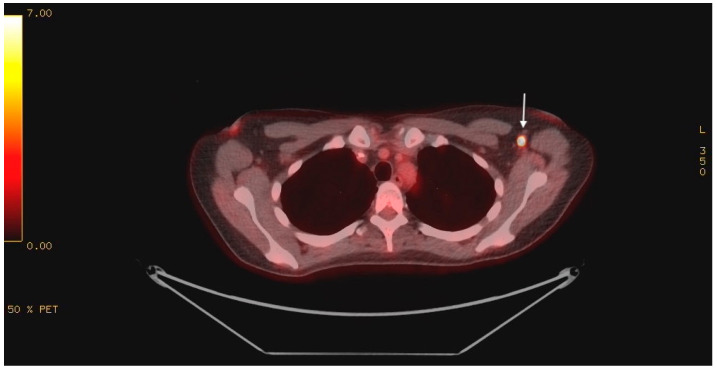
PET scan showing intense 18-FDG uptake in the left axilla (indicated by white arrow).
